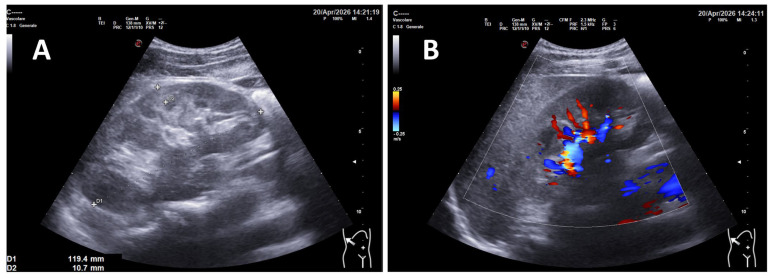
Grayscale and Doppler acquisition sequence. (A) Longitudinal grayscale image showing maximum bipolar diameter and cortical thickness measurement, followed by (B) color Doppler identification of an interlobar artery adjacent to a medullary pyramid (region of interest, ROI).
